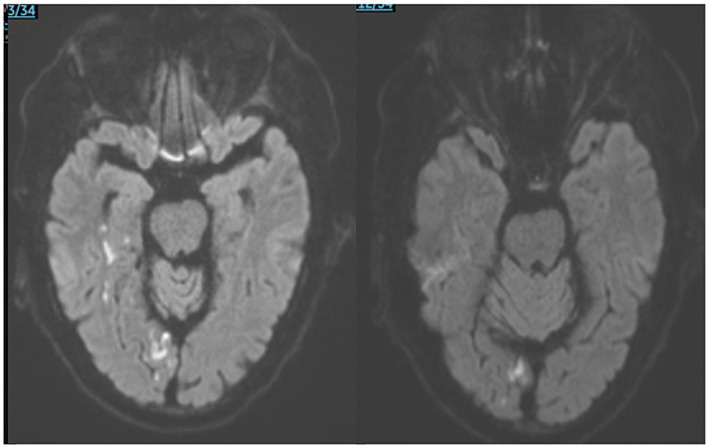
Axial diffusion-weighted magnetic resonance imaging (DWI) demonstrating an acute ischemic infarction involving the right occipital lobe within the posterior cerebral artery territory. The two slices demonstrate the extent of the infarction.
